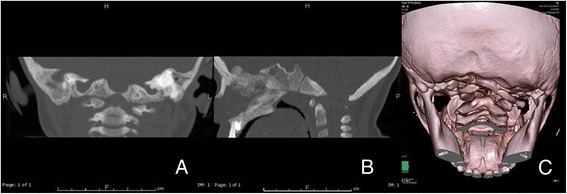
a Coronal CT multiplanar reconstruction showing asymmetric occipital condyles aspects. b Sagittal CT multiplanar reconstruction shows clivus platybasic aspect. c 3D reconstruction (bone volume rendering) showing dysmorphic aspects of cranio-cervical junction
